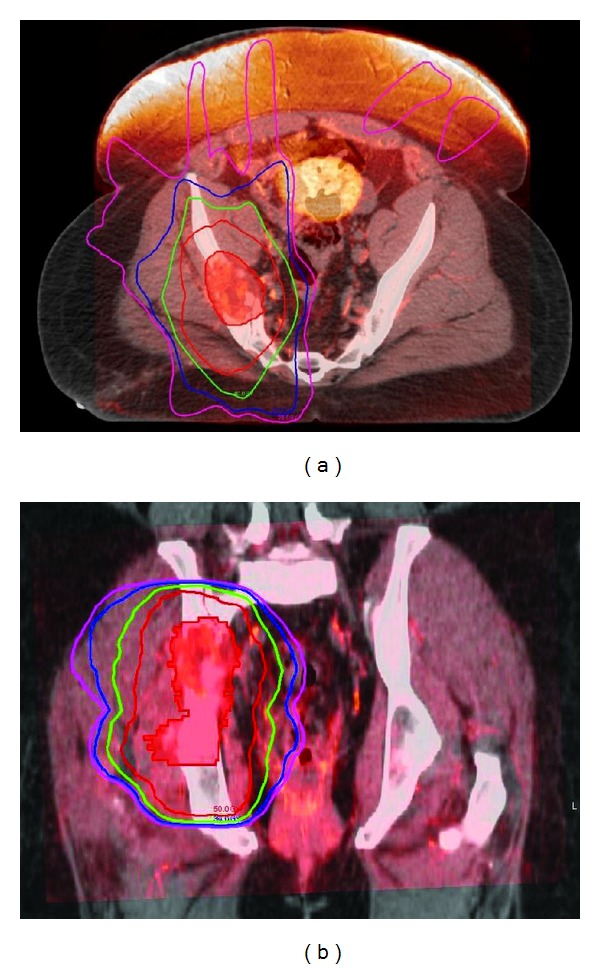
Fusion of the planning CT and the preoperative T1-weighted postcontrast MRI utilized to outline the clinical targeting volume (red colorwash) and to develop an Intensity Modulated Radiation Therapy plan depicting the isodose profiles for 50 Gray (red), 40 Gray (green), 30 Gray (blue), and 25 Gray (purple) in the axial (a) and the coronal (b) planes.
